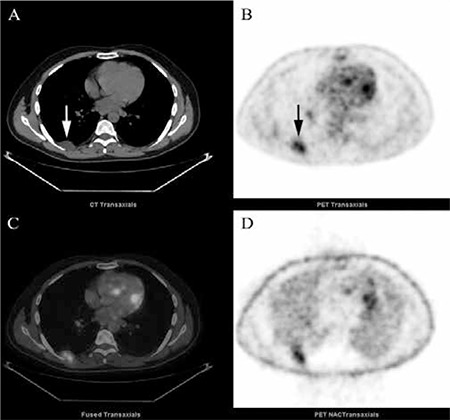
Transaxial (a) CT, (b) PET, (c) PET/CT fusion and (d) NAC PET images. A second mass lesion of the posterior chest wall in the 7th intercostal space showing moderately increased FDG uptake (SUVmax: 5.6) and 30x17 mm in size is detected (arrows).
